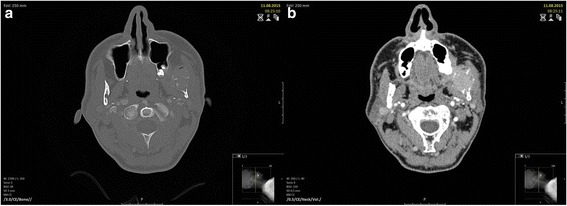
a b preoperative CT scan (soft tissue and bone window) showing the tumouros lesion with a bone defect in the left mandible
